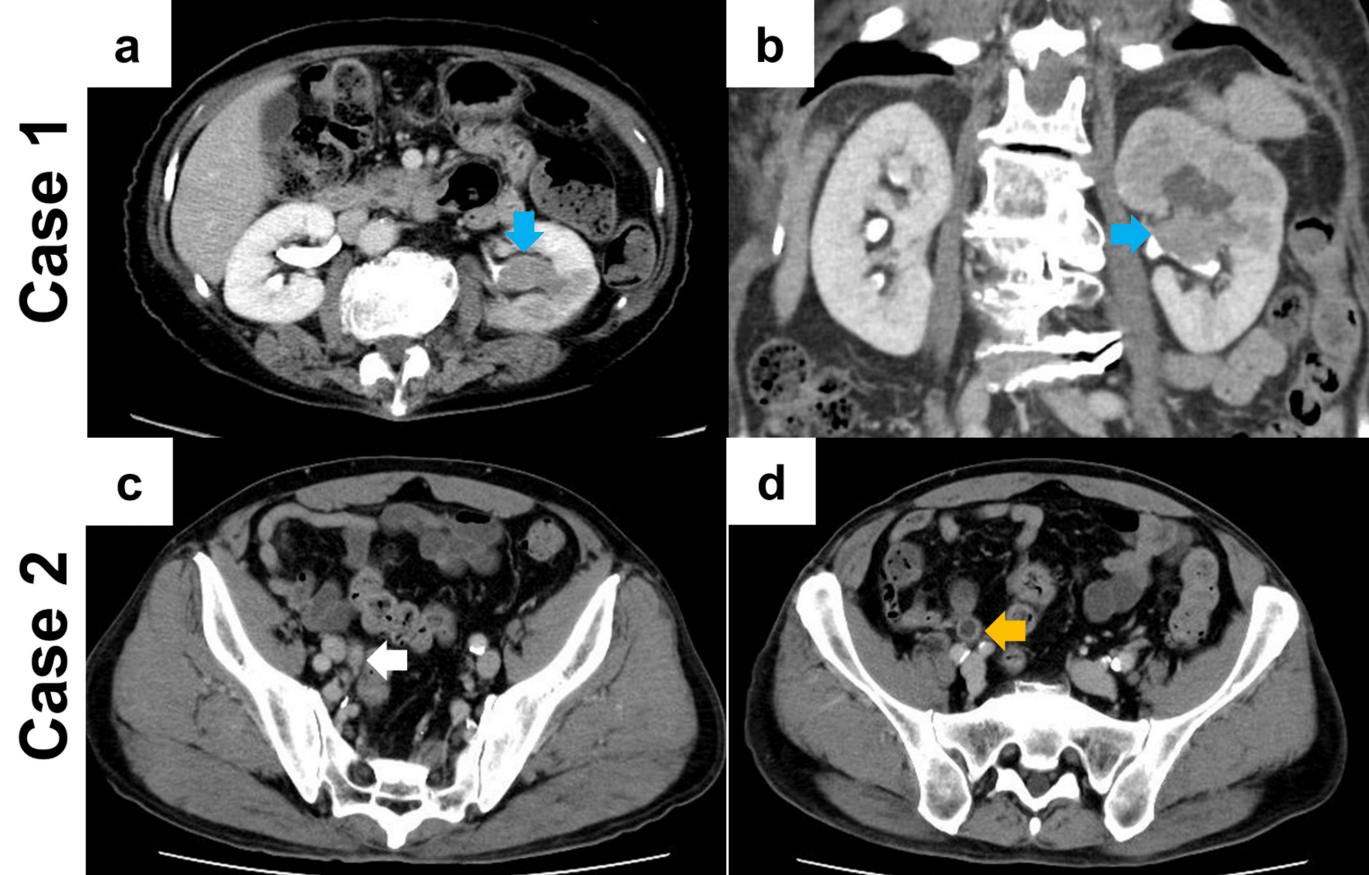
Computed tomography in Cases 1 and 2 (a and b): Computed tomography (CT) demonstrating a left renal pelvic tumor enhanced by contrast medium, with a diameter of 30 mm in Case 1. There is suspicion of parenchymal invasion (a: axial section, b: coronal section). The blue arrow indicates a left renal pelvic tumor (c and d): CT demonstrating a right ureteral tumor (white arrow) exhibiting contrast enhancement near the right iliac vessel and a thickened ureteral wall (orange arrow) on its cephalic side in Case 2.
